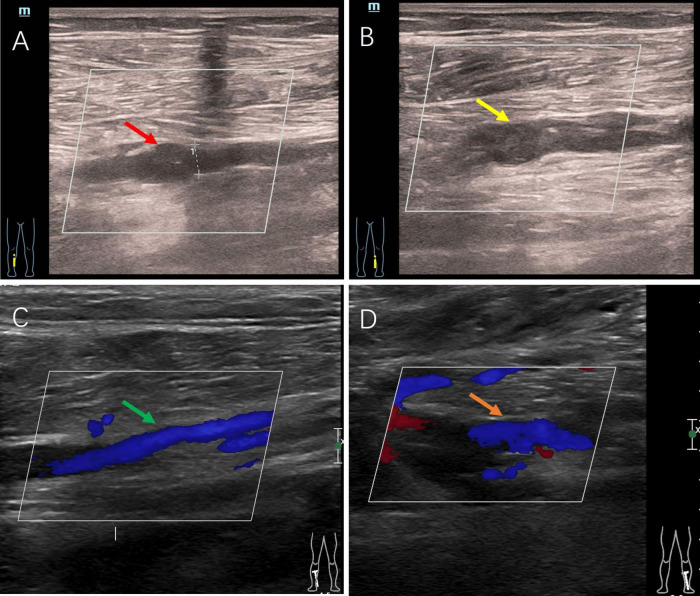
Venous ultrasound of the legs on admission showing intermuscular venous thrombosis in the right calf ((A), red arrows); and left calf (B), yellow arrows). Follow-up venous ultrasound of the legs after 2 months of rivaroxaban therapy revealed complete resolution of the intermuscular vein thrombosis (right calf, (C), green arrows; and left calf, (D), orange arrows).
